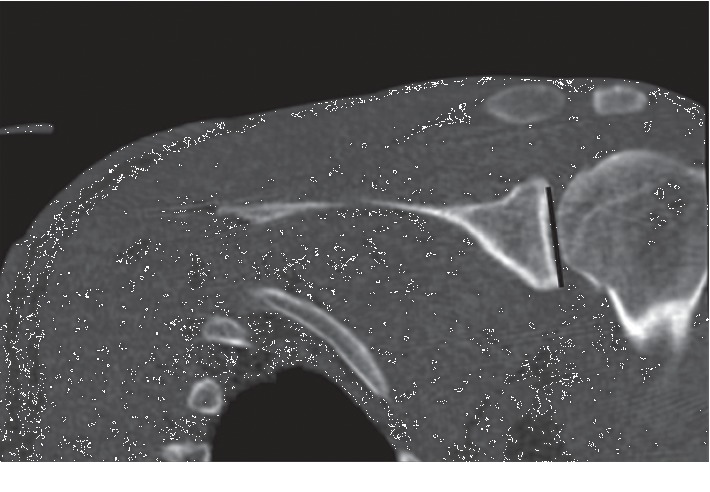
Measurement of the SI diameter (height) of the glenoid on the coronal CT image.
